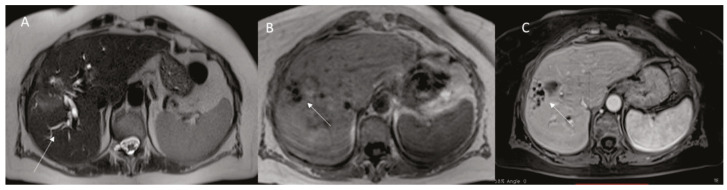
Woman 63 year at 1-month follow-up after RFA of liver metastasis. MRI assessment ((A): HASTE T2-W sequence; (B): in phase T1-W sequence and (C): porta phase of contrast study), ablated zone with biliary tree damage (arrow).
